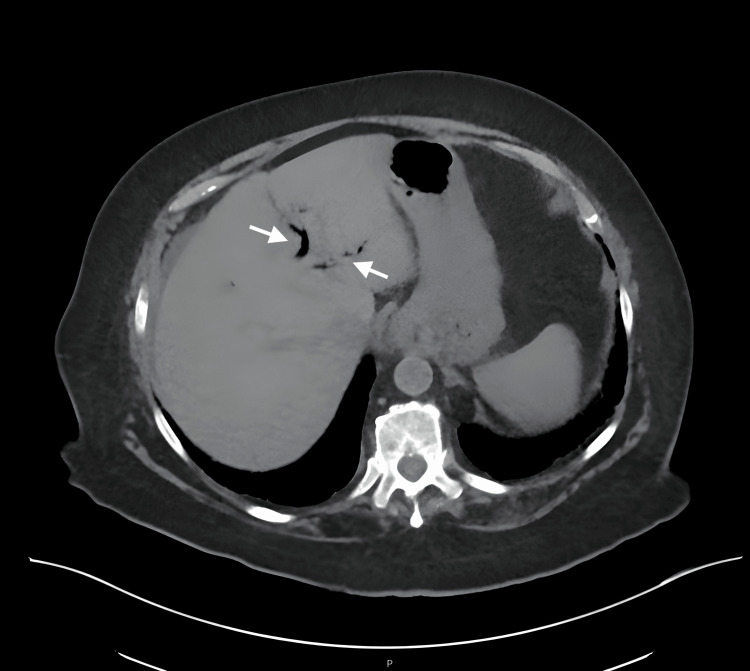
Anteroposterior (AP) CT imaging in the axial plane demonstrating subtle air within the biliary tree (arrows)
